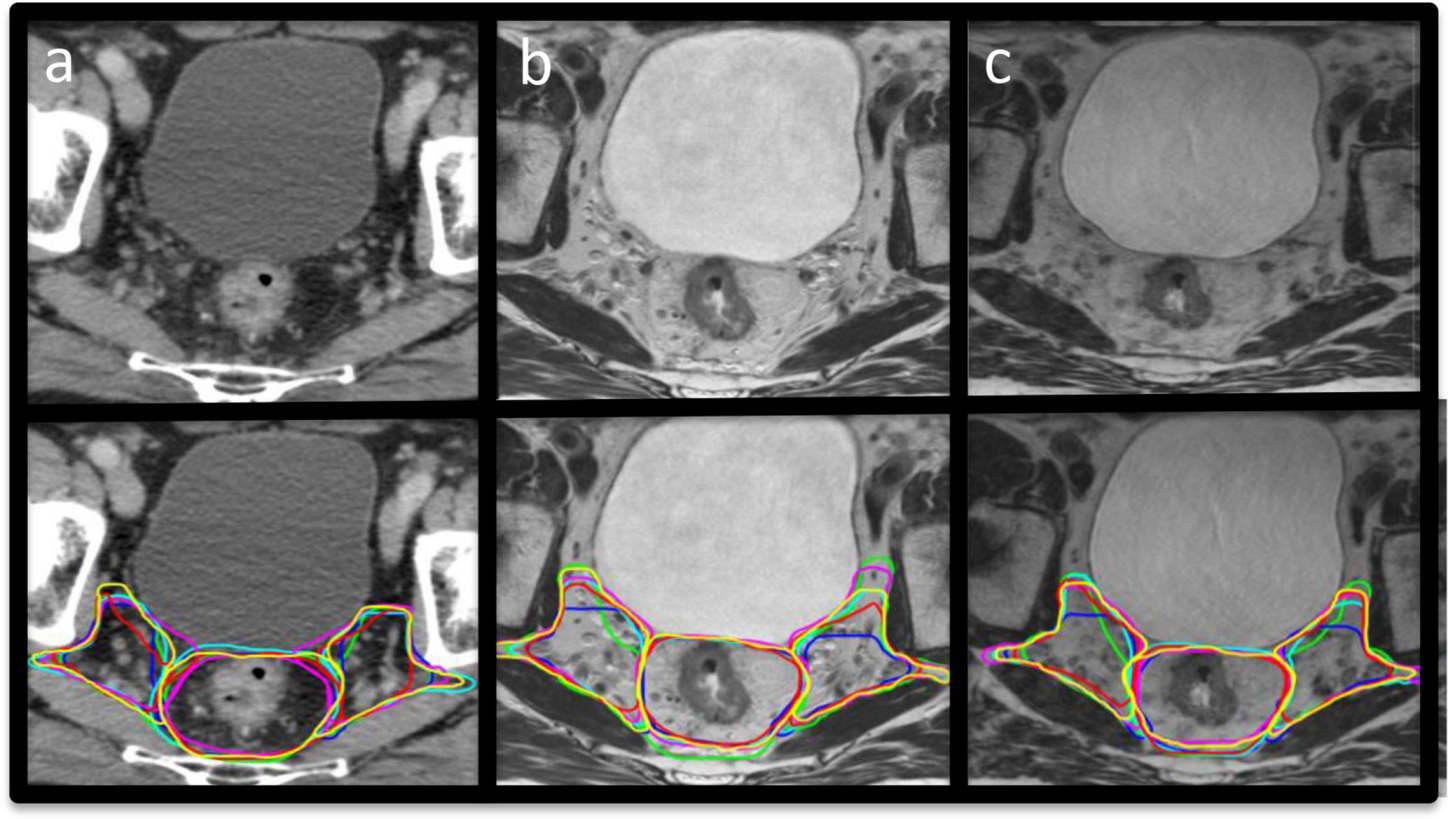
Example images with and without observer CTV contours at week 0. (a) CT, (b) 2D-MRI and (c) 3D-MRI. CTV, clinical target volume.
